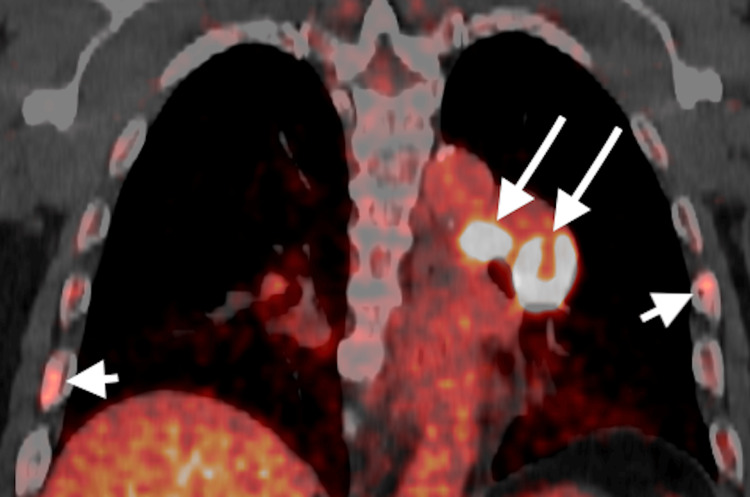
Reformatted coronal PET image through the chest. Demonstrates multiple FDG-avid foci involving the left perihilar region (long arrows) and bilateral ribs (short arrows).
